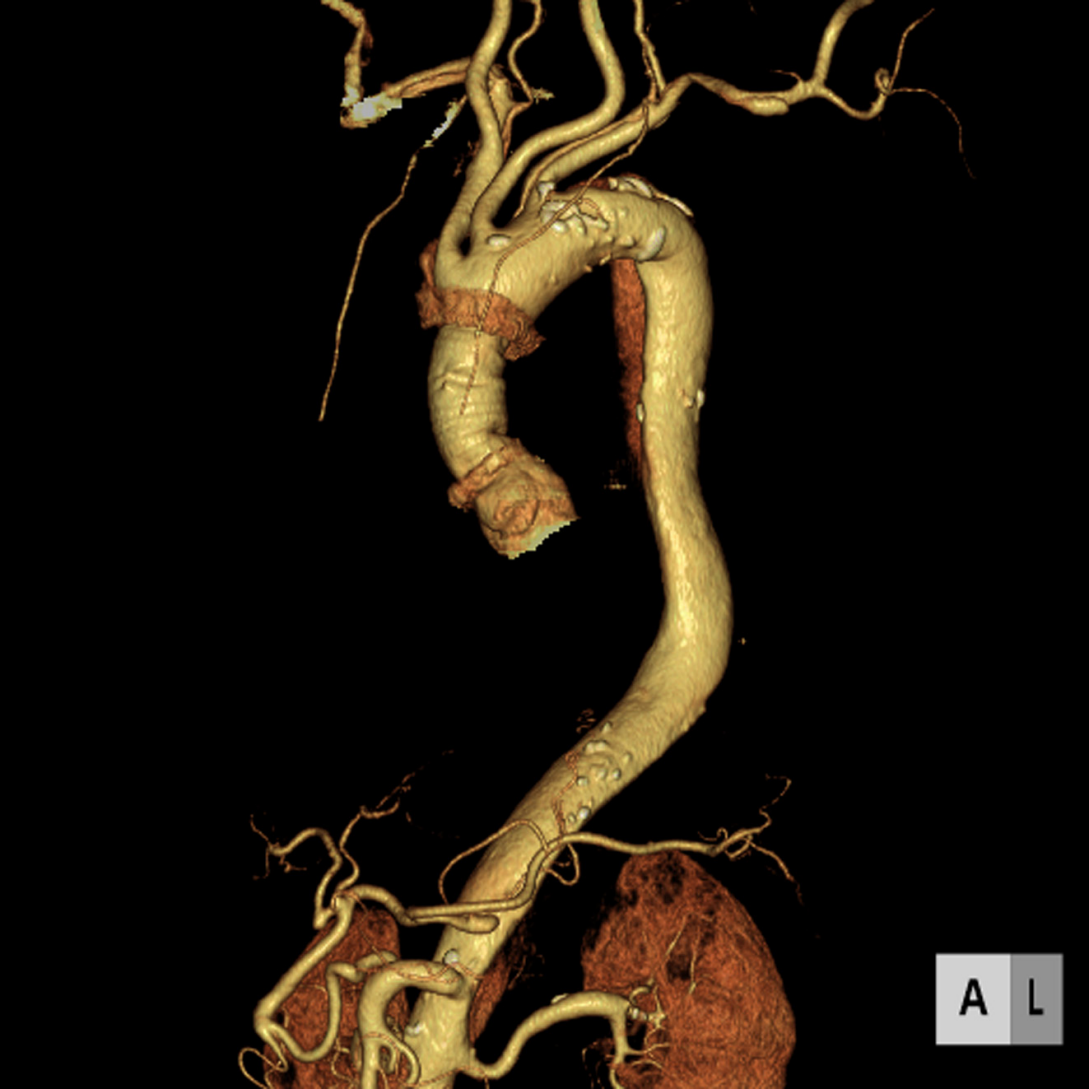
3D computed tomography imaging showing ascending aorta replaced with an artificial graft.
